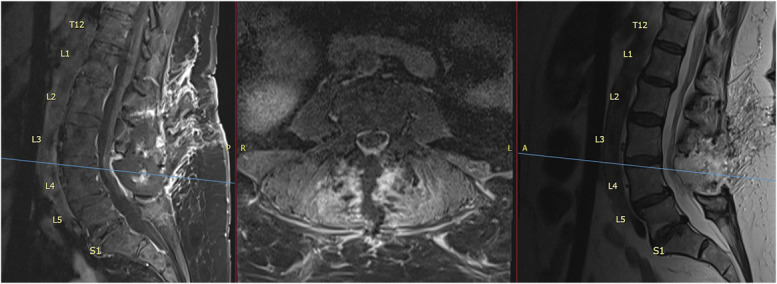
Postoperative MRI—Case 1. The sagittal T1 weighted MRI with contrast (left), axial T1-weighted with contrast (center), and sagittal T2 (right) images demonstrate complete resection of the posterior epidural lesion with restoration of the thecal sac at L4-5 and confirm the absence of the previously noted facet-associated epidural mass.
